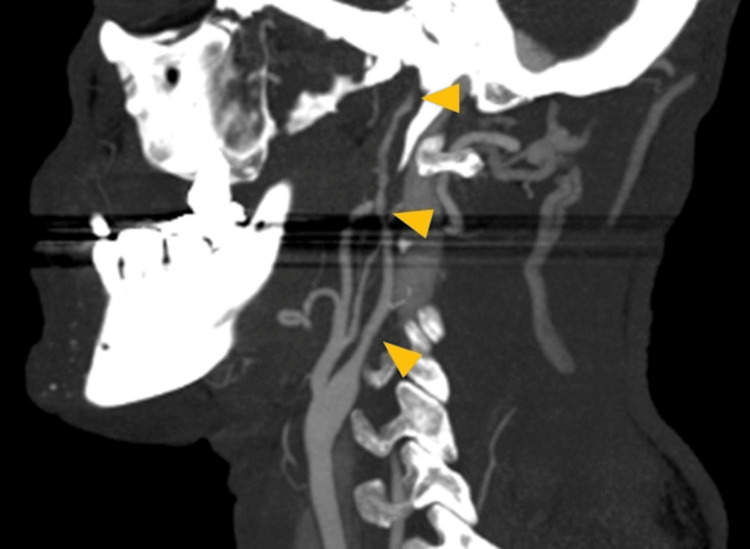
Sagittal CT angiography of the neck arteries showing irregular and filiform cervical segment of the right internal carotid artery consisted with dissection
